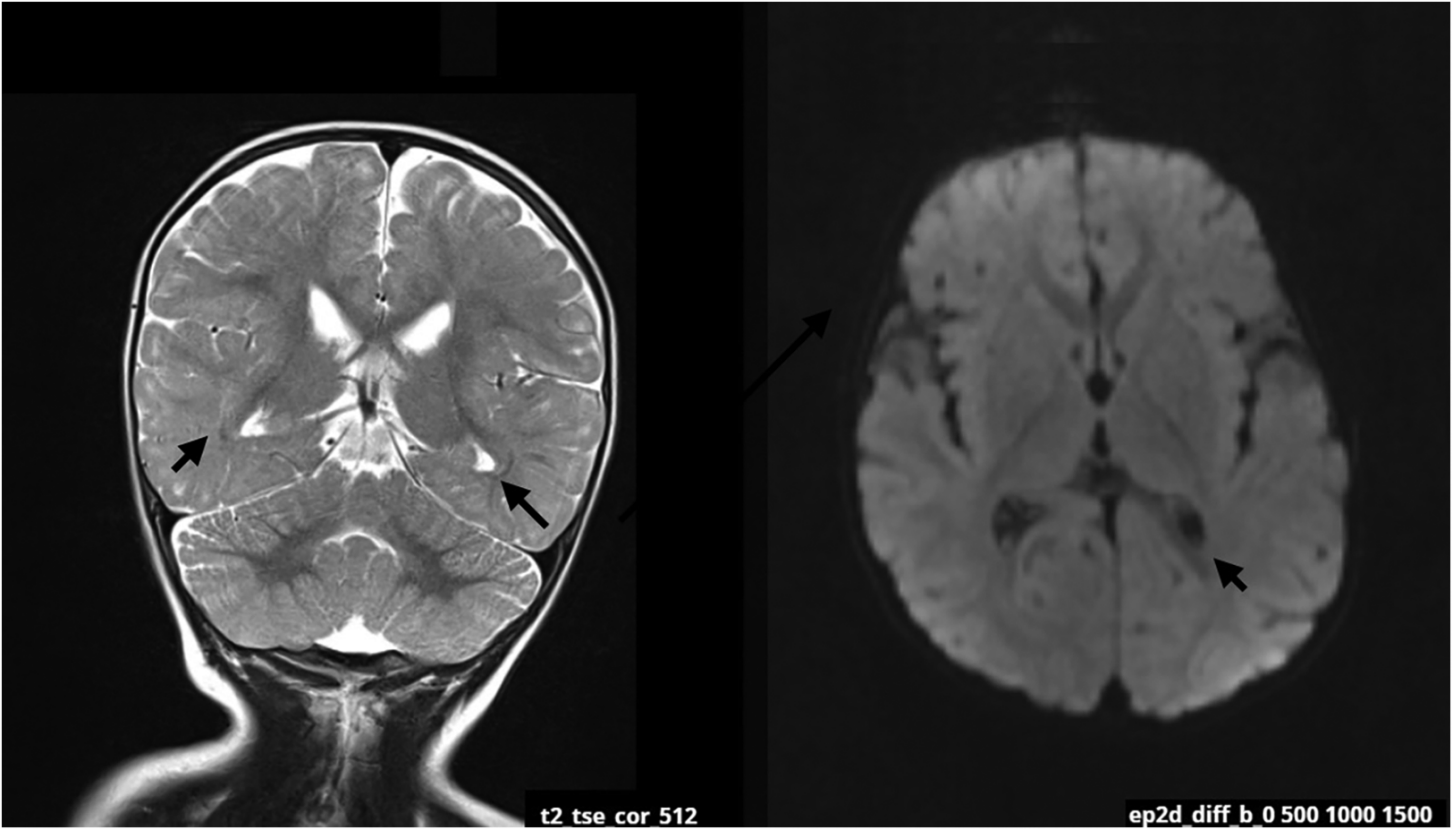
MRI scan at the age of 14 months. The arrows are pointing zones of increased T2/FLAIR signal in the white matter around the occipital horns of the lateral ventricles. The changes may be related to delayed myelination of this region.
